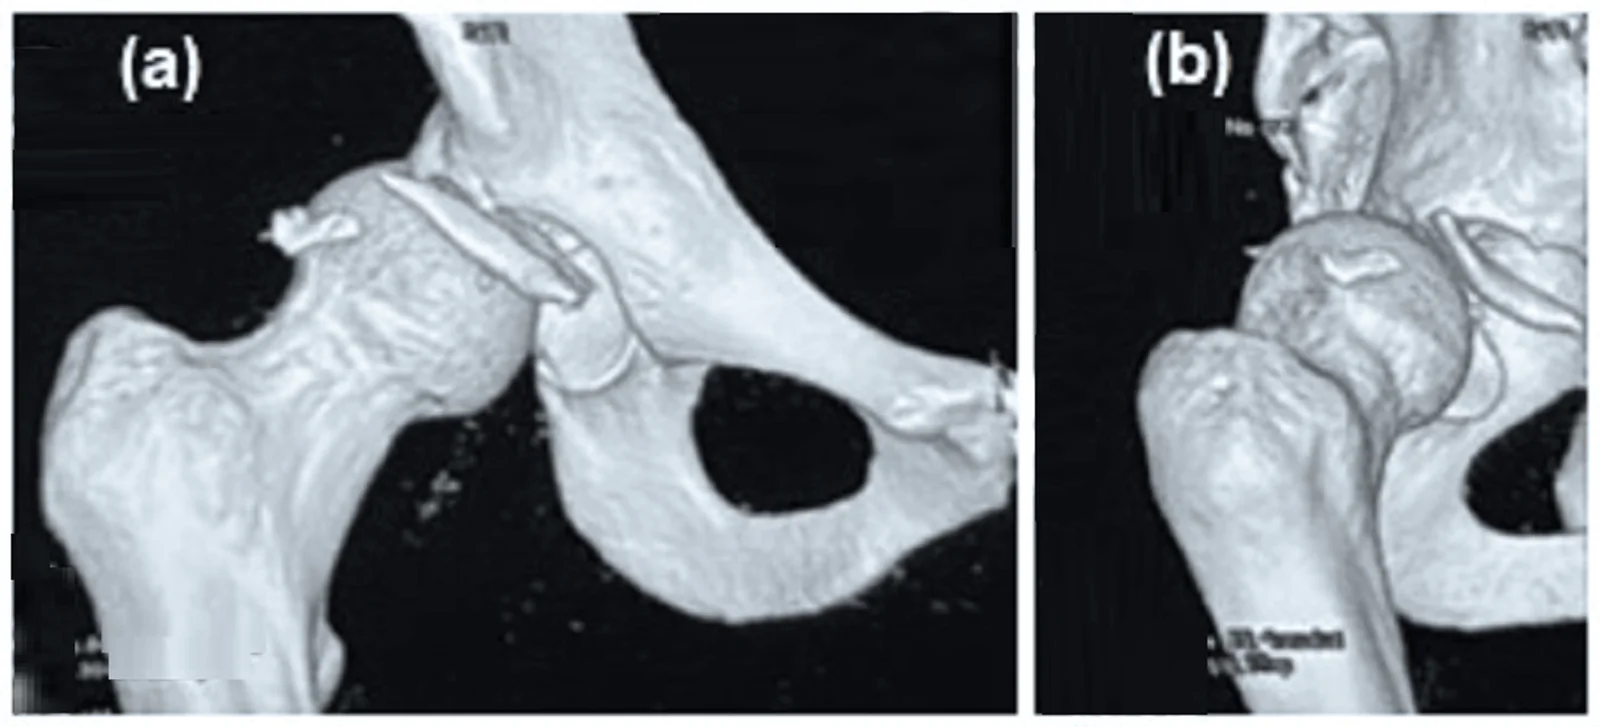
Case 3: Preoperative evaluation. (a) Three-dimensional CT reconstruction demonstrating posterior hip dislocation associated with a posterior wall acetabular fracture. (b) Three-dimensional CT reconstruction showing the incarcerated posterior wall fragment within the hip joint, preventing concentric reduction.
